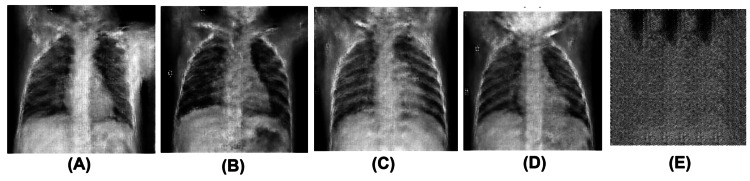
Fake chest X-ray images generated by the generator model. These images display structural features in real chest X-rays, such as the rib cage, symmetrical lung fields, diaphragm, left-sided heart, shoulders, arms, and neck. Image (A) shows a raised right arm and a lowered left arm, with a blurred and faint gastric bubble and trachea. Image (B) reveals a visible gastric bubble, a blurred tracheal shadow, and bilateral clavicles. Image (C) is notable for its remarkable heart size. Image (D) features a prominent blurred head shadow, resembling a pediatric chest X-ray (CXR). There are noticeable imperfections, such as slight blurring and artifacts throughout the images, which are common in generative adversarial network-generated outputs. Images (A) and (B) are CXR images generated using the generator model after 500 training epochs, while images (C) and (D) are images generated using the generator model after 200 training epochs. Image (E) produced no meaningful images after 500 epochs.
